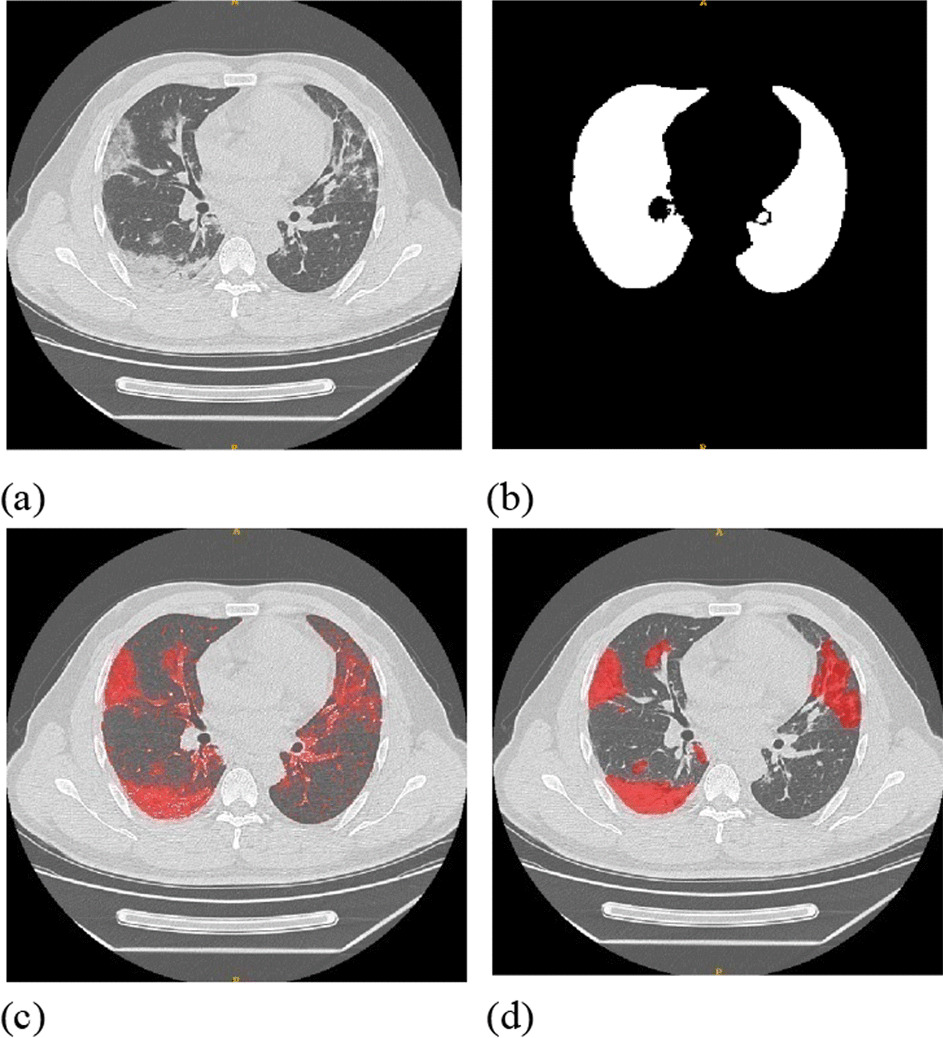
Typical Hu-based pseudo-labeled and manually labeled CT images: a original CT image with pneumonia; b generated mask of intact lung; c pseudo-labeled (Hu_[− 750,50]_); d manually annotated by radiologists
